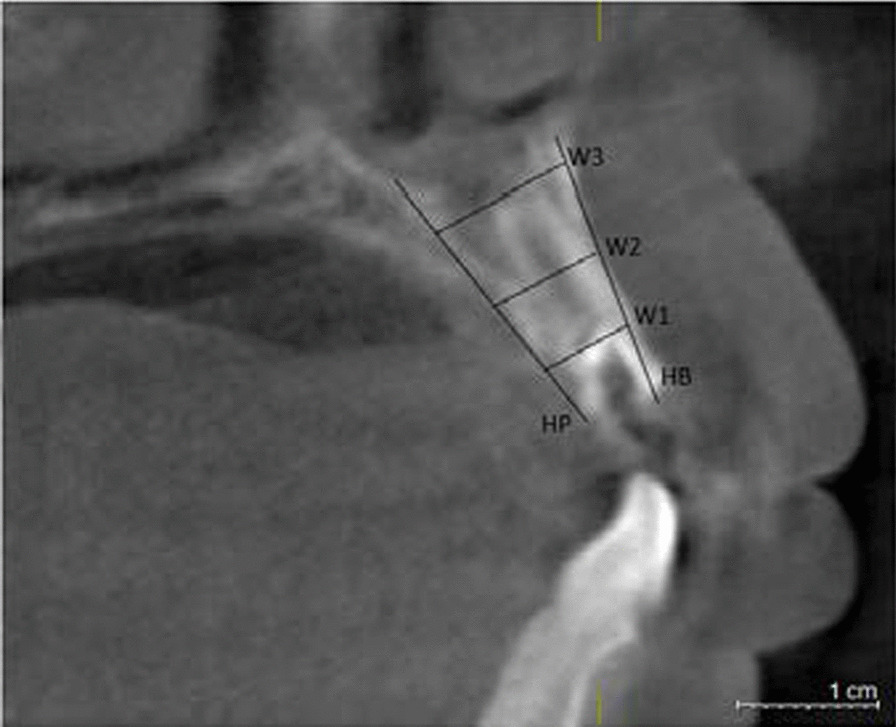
The radiographic landmarks used for measurement of bone width and bone height on CBCT images
